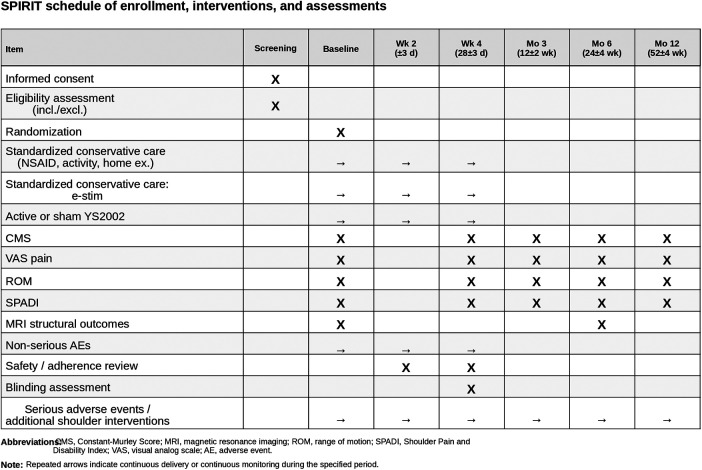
SPIRIT schedule of enrollment, interventions, and assessments. Schedule of screening, baseline assessment, allocation, intervention delivery, safety and adherence review, primary endpoint assessment at Week 4, and follow-up assessments through Month 12. CMS, Constant-Murley Score; MRI, magnetic resonance imaging; ROM, range of motion; SPADI, Shoulder Pain and Disability Index; VAS, visual analog scale.
